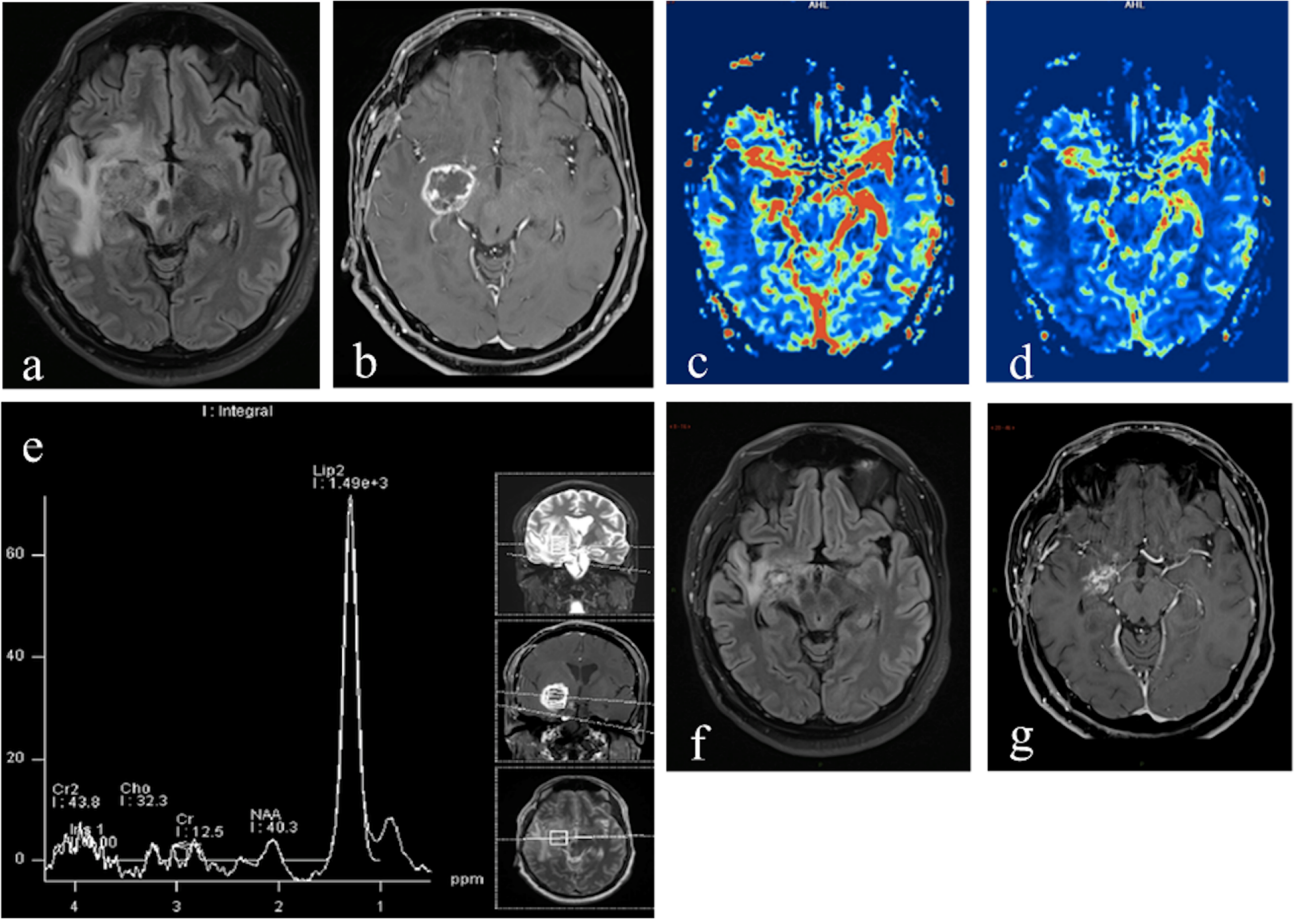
MRI performed 13 months after proton therapy for clival chondrosarcoma. Axial fluid-attenuated inversion recovery (FLAIR) image (a) shows a disomogeneous area of altered signal, with hypo- and hyperintense components, located in the right hippocampal region and surrounded by extensive edema involving subcortical temporal and frontoinsular white matter, the cortico-spinal tract and causing mass effect on the right cerebral peduncle. Axial postcontrast T1-weighted image (b) shows peripheral and disomogenous enhancement, with “soap bubble” like pattern. rCBV (c) and CBF (d) maps show no hyperperfusion in the enhancing area. MRS-short TE (e) reveals the presence of high lipid and lactate peak with general decrease of other metabolites, highly suggestive for radiation necrosis. Control MRI performed in the same patient after 3 months; no intercurrent therapies. Axial FLAIR image (f) shows an evident decrease of the vasogenic edema, confined to temporal lobe. Axial T1-post contrast-weighted image (g) shows reduction in size of the enhancing lesion
